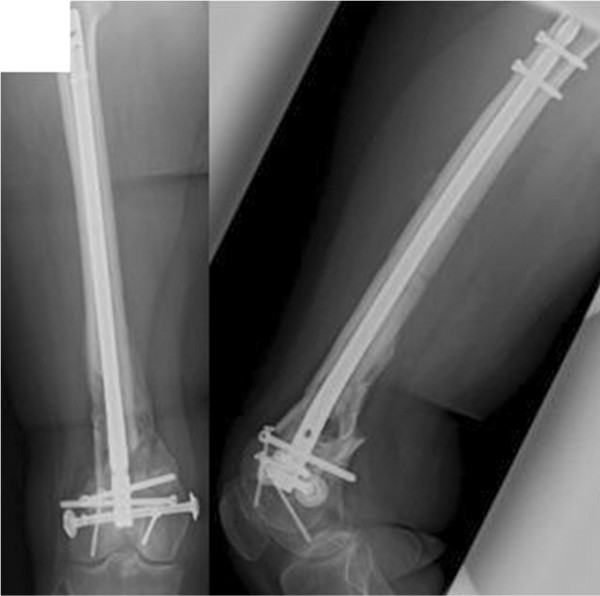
Postoperative imaging (X-ray).
